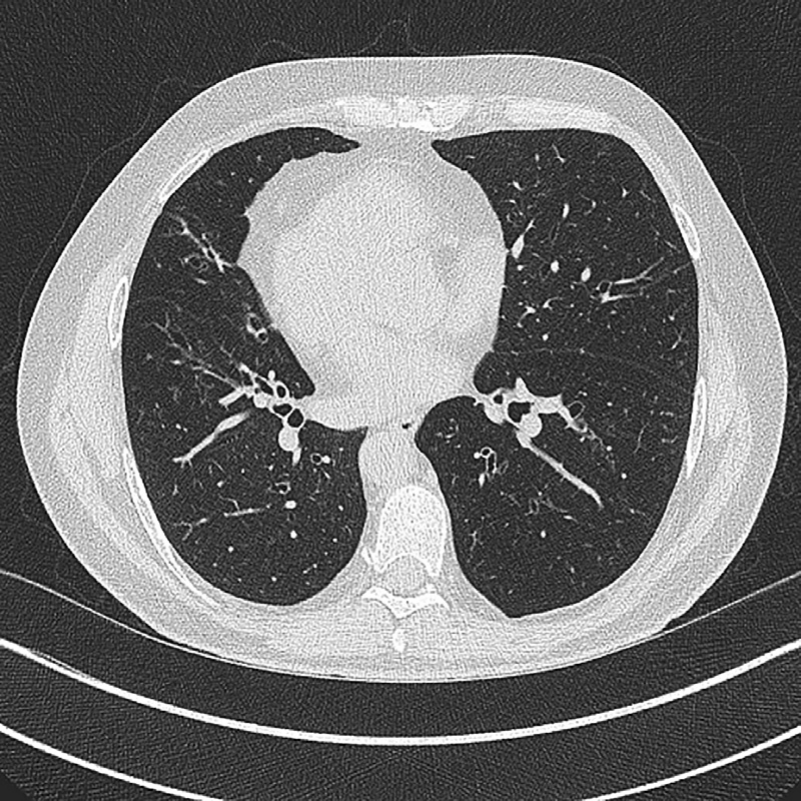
An axial section of a high-resolution chest CT scan demonstrating bronchiectasis and dextrocardia in an individual with PCD.
